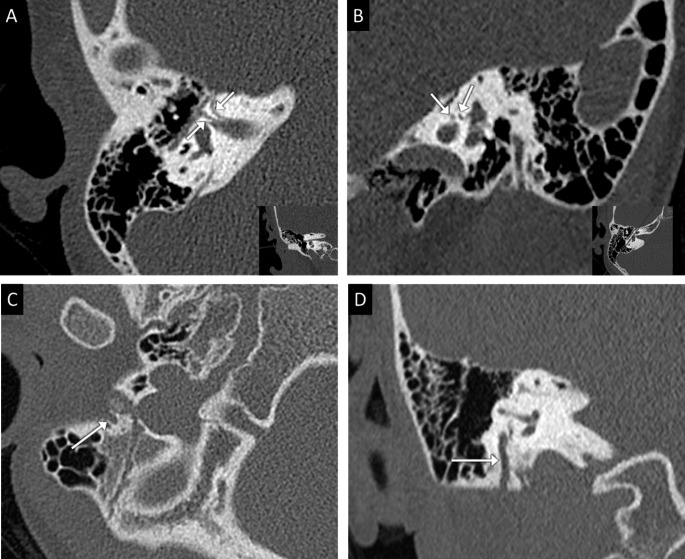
Computed tomography focused on the right intratemporal facial nerve. (a) axial oblique view, (b) view perpendicular to the labyrinthine segment: Right facial nerve bifurcation on its labyrinthine segment (arrows). (c) axial view, (d) coronal view: short and anterior mastoid segment of the facial nerve opened anteriorly between the mastoid apophysis and the glenoid cavity (arrows)
